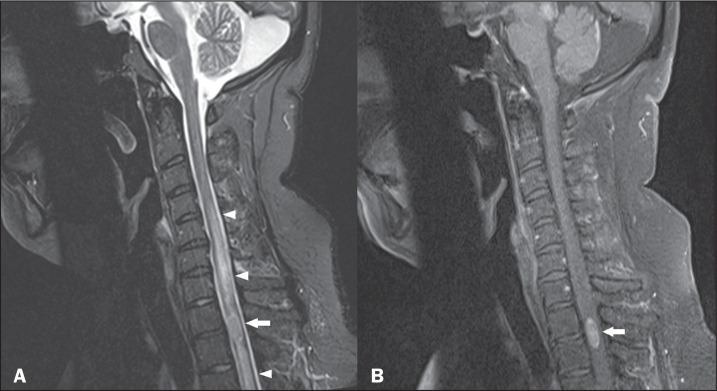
Spinal cord metastasis of clear cell renal carcinoma. Sagittal MRI scans.
